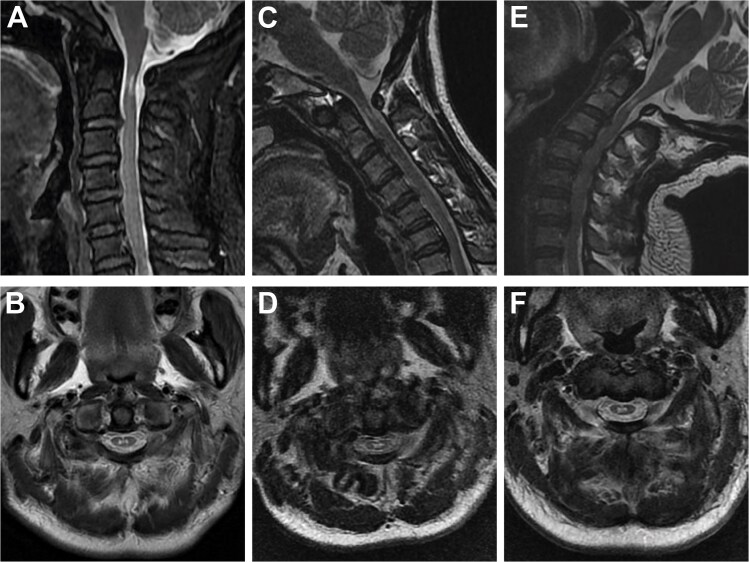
Sagittal and axial T2-weighted MRI of the cervical spine. (A, B) Supine neutral position. (C, D) Supine neck flexion position. (E, F) Supine neck extension position.
